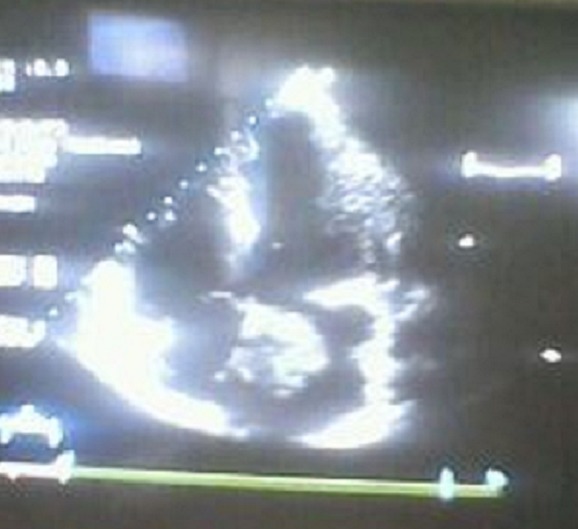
Vue parasternale myxome en diastole
